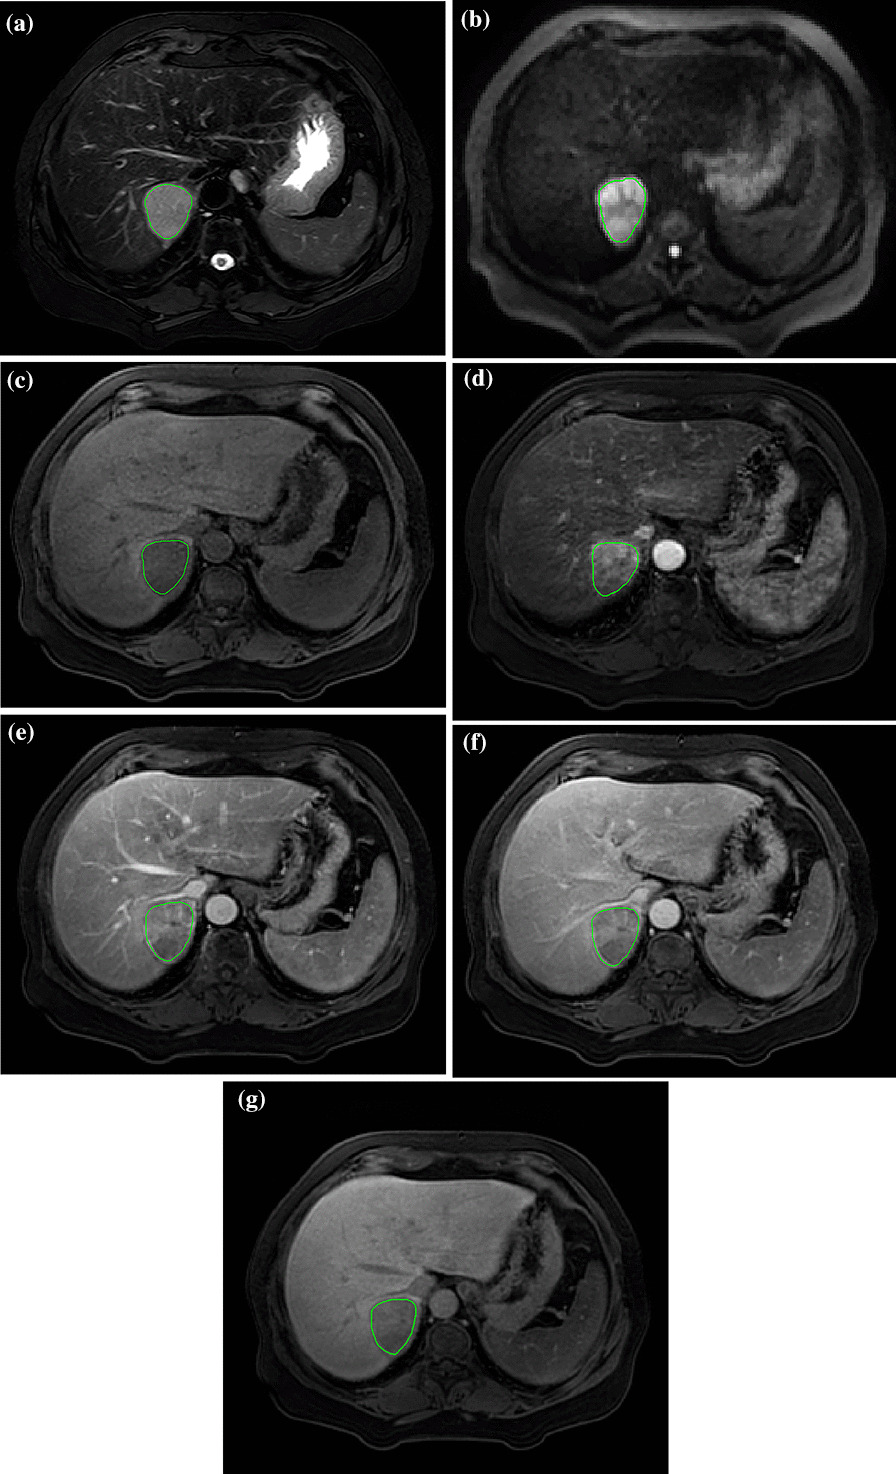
The delineation of ROI on T2WI (a), DWI (b), T1WI (c), AP (d), PP (e), TP (f) and HBP (g). The ROI was drawn as close to the margin of the tumour as possible but within the margin on the largest section
